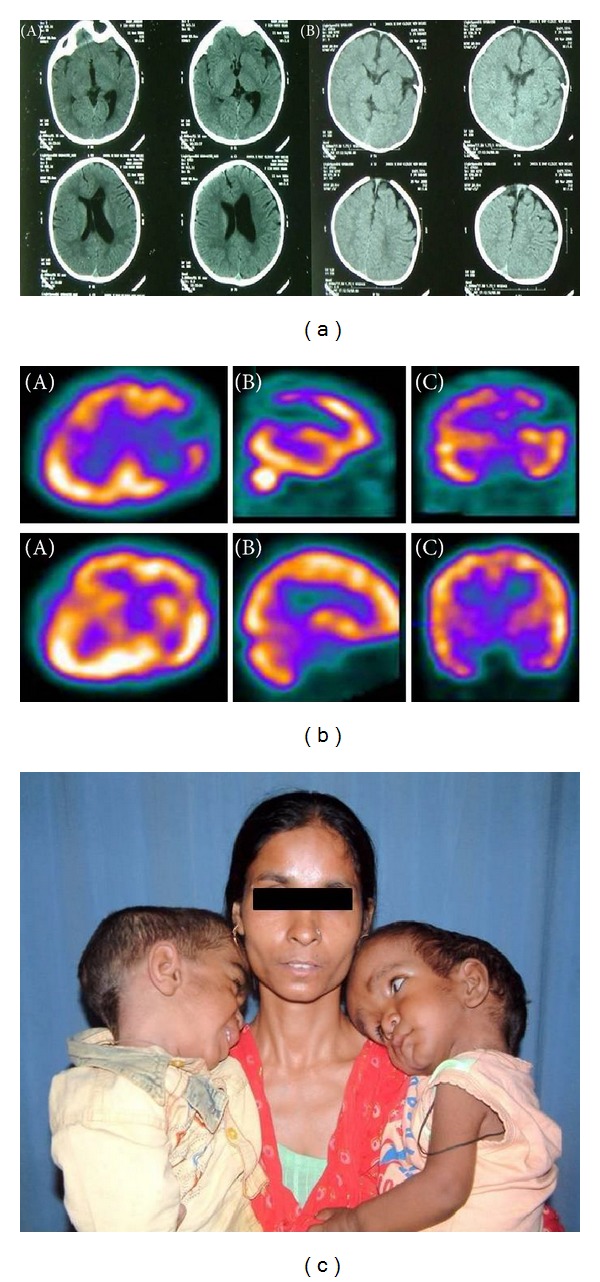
(a) Preoperative axial noncontrast CT (NCCT) skull images showing affected sulcal spaces of left frontoparietal lobe at the convexity and ventriculomegaly (A). Postoperative images showing decreased ventricular size and (B). (b) ^99m^Tc-ECD brain SPECT preoperatively showing absent radiotracer uptake involving left frontoparietal lobe suggestive of hypoperfusion, upper panel images axial (A), sagittal (B), and coronal (C), and postoperatively showing increased radiotracer uptake involving left frontoparietal lobe suggestive of hyperperfusion, lower panel images axial (A), sagittal (B), and coronal (C). (c) The preoperative clinical image of twin patients. Note the enlarged skull secondary to craniosynostoses and hydrocephalus in the patient on right arm of the mother.
